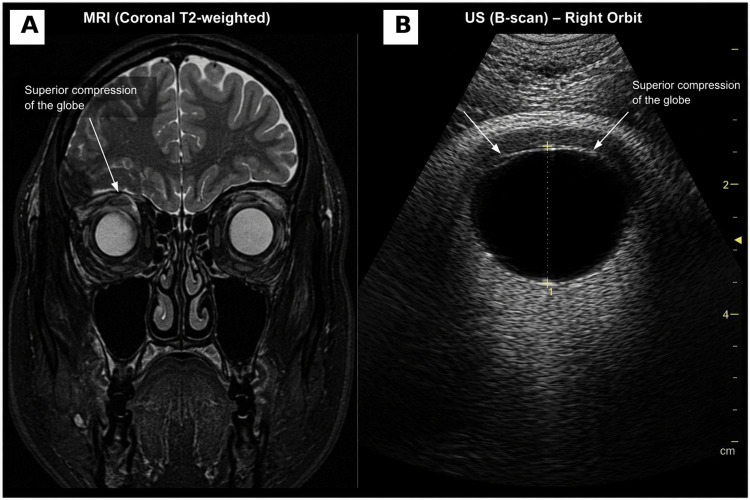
Orbital imaging demonstrating superior compression of the right globe (A) Coronal T2-weighted MRI showing superior orbital soft tissue causing downward mass effect on the globe. (B) B-scan ultrasonography demonstrating flattening of the superior scleral contour consistent with external compression by orbital disease MRI: magnetic resonance imaging
